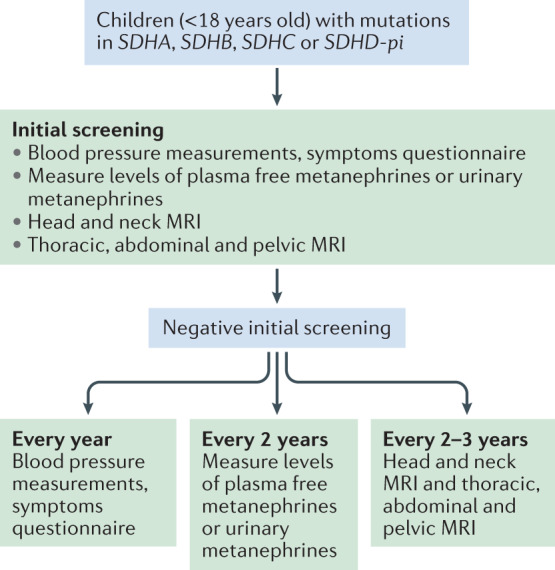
Screening and follow-up proposed during childhood. An initial tumour screening should be performed after the discovery of an *SDHA*, *SDHB*, *SDHC* or *SDHD-pi* mutation relying on blood pressure measurements, a symptoms or signs questionnaire, assessment of metanephrines in plasma or urine, and imaging work-up by MRI of head and neck, thorax, abdomen and pelvis. Even after an initial negative work-up, all asymptomatic mutation carriers should be clinically followed up every year, by biochemical assessments every 2 years and by MRI every 2–3 years.
